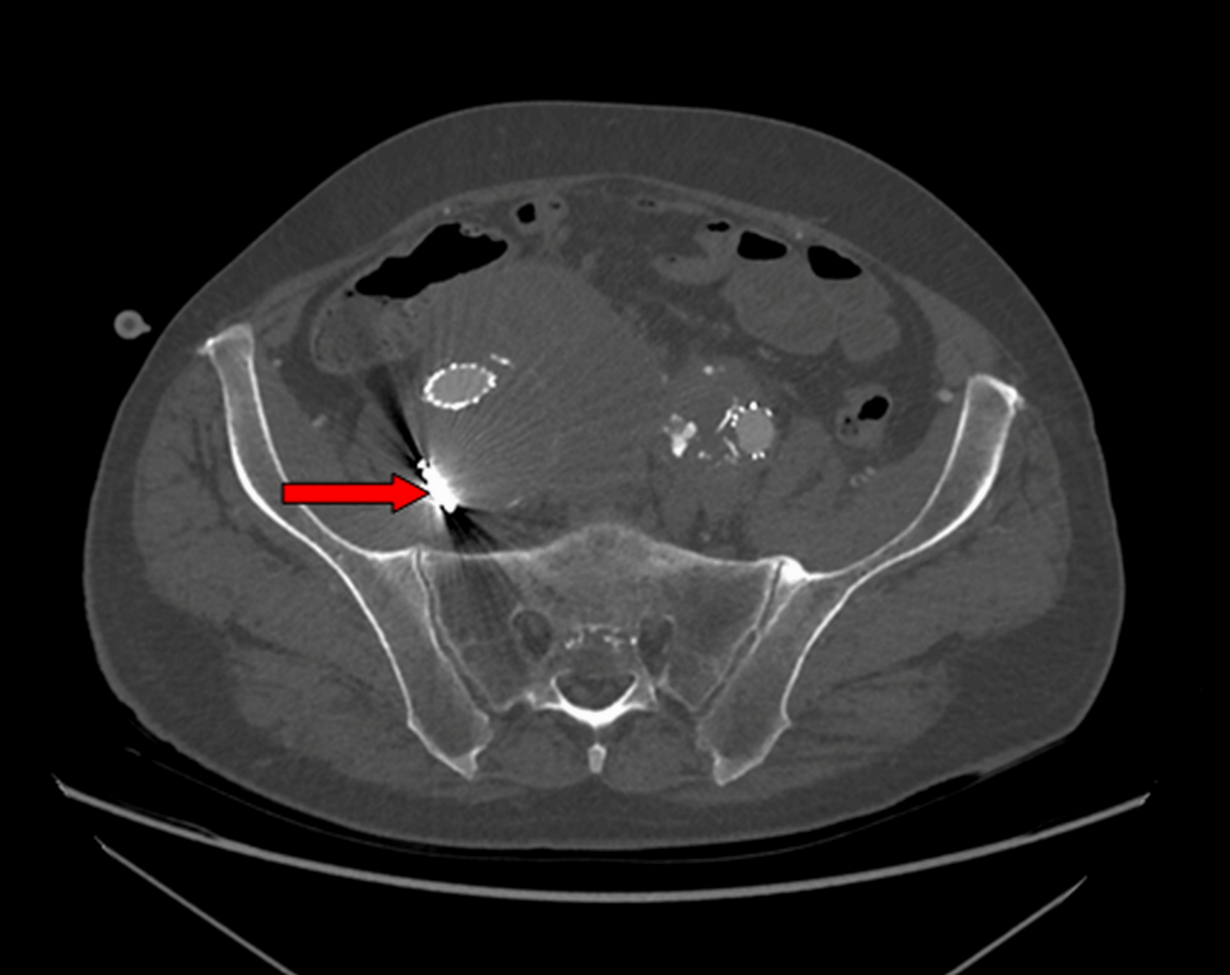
CT showed status post endovascular recanalization of renal artery stents and embolization of the right internal iliac artery (red arrow).
